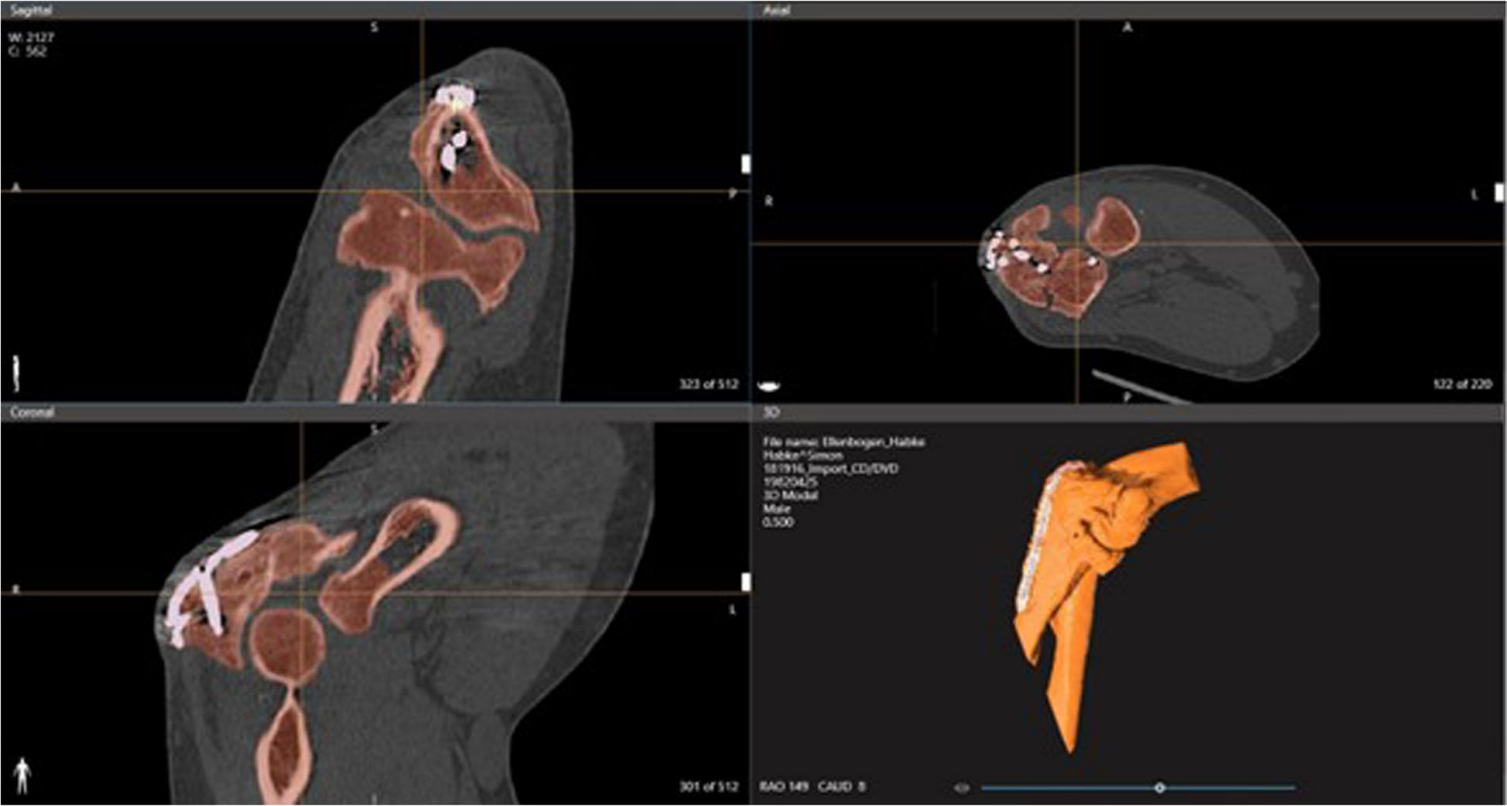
Segmentation of the anatomical structures with the help of CT-images
